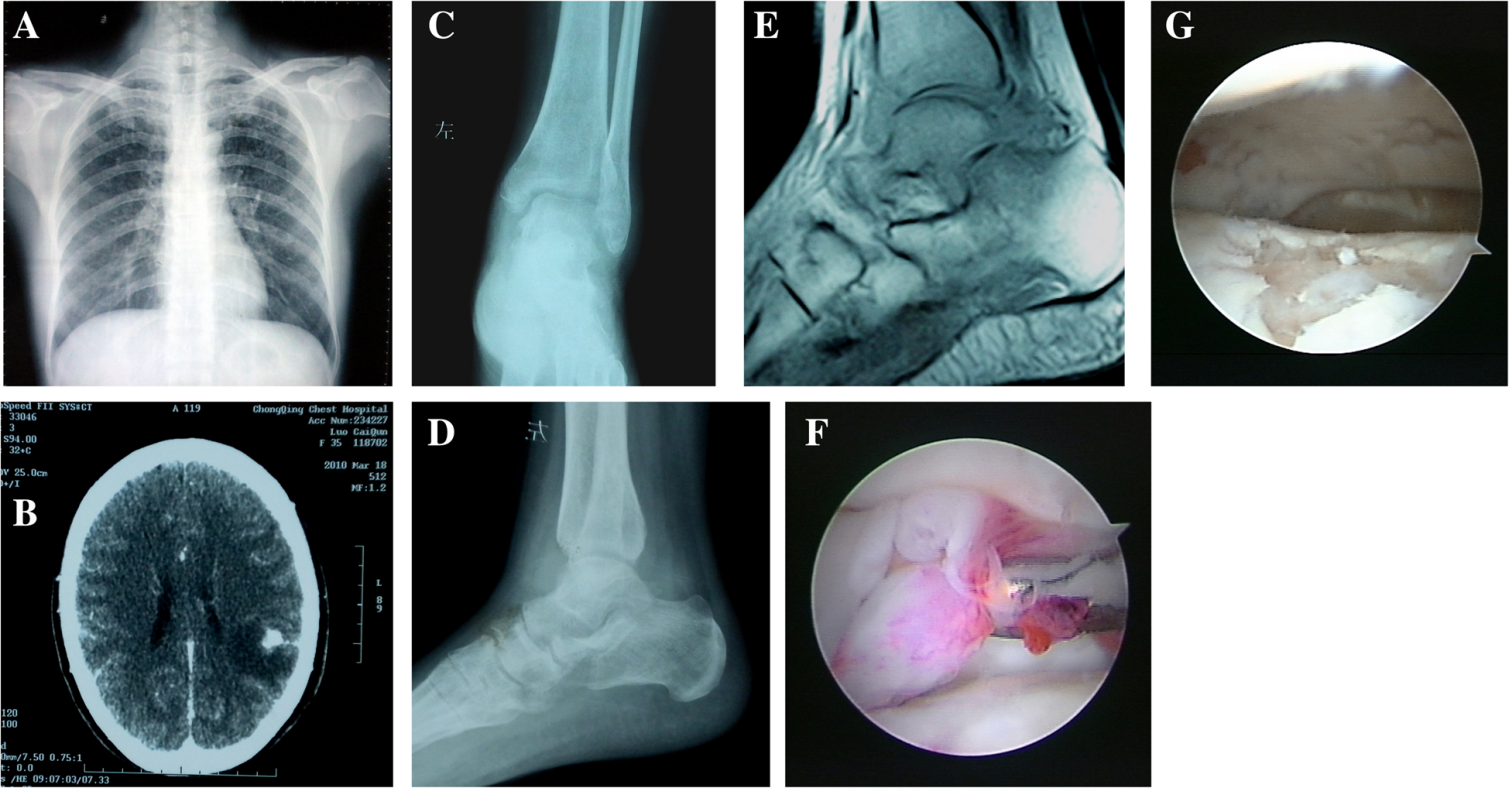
a Chest radiograph suggesting tuberculosis. b Cranium CT showing intracranial lesions. c Preoperative radiograph suggesting ankle diffuse osteoporosis (anteroposterior view). d Preoperative radiograph suggesting ankle diffuse osteoporosis (lateral view). e Preoperative MRI suggesting inflammatory tissue of ankle and subtalar joint. f Hyperplasia of synovial tissue under ankle arthroscope. g Cartilage defects can be seen after debridement, with the residual cartilage remaining stable
